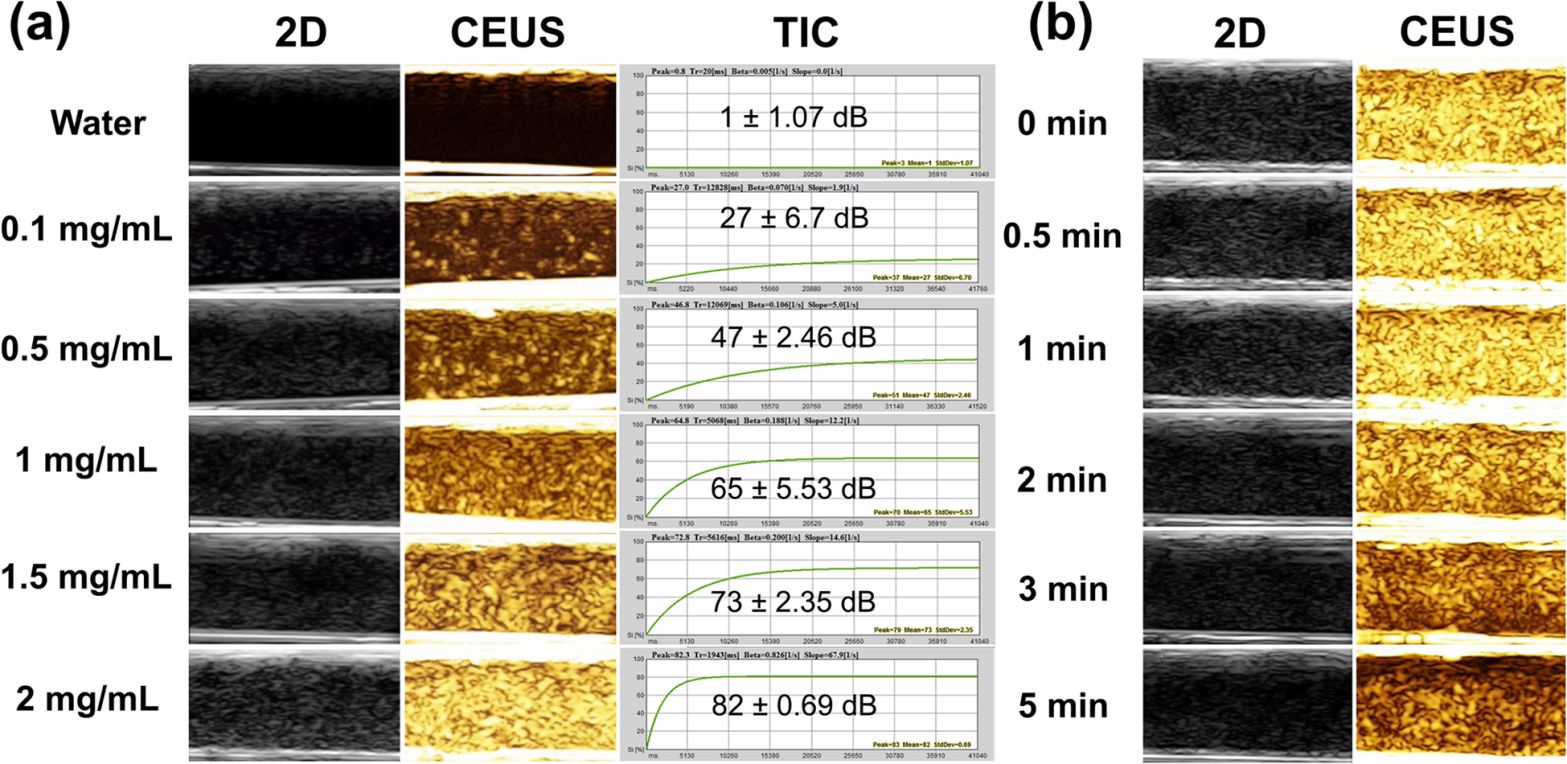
a In vitro two-dimensional (2D) gray-scale ultrasound (US) and contrast-enhanced ultrasound (CEUS) images and the time-intensity curve (TIC) of Her2-GPH NPs under different concentrations (0, 0.1, 1, 1.5, 2 mg/mL). b In vitro US images of Her2-GPH NPs (2 mg/mL) with time elapsing
